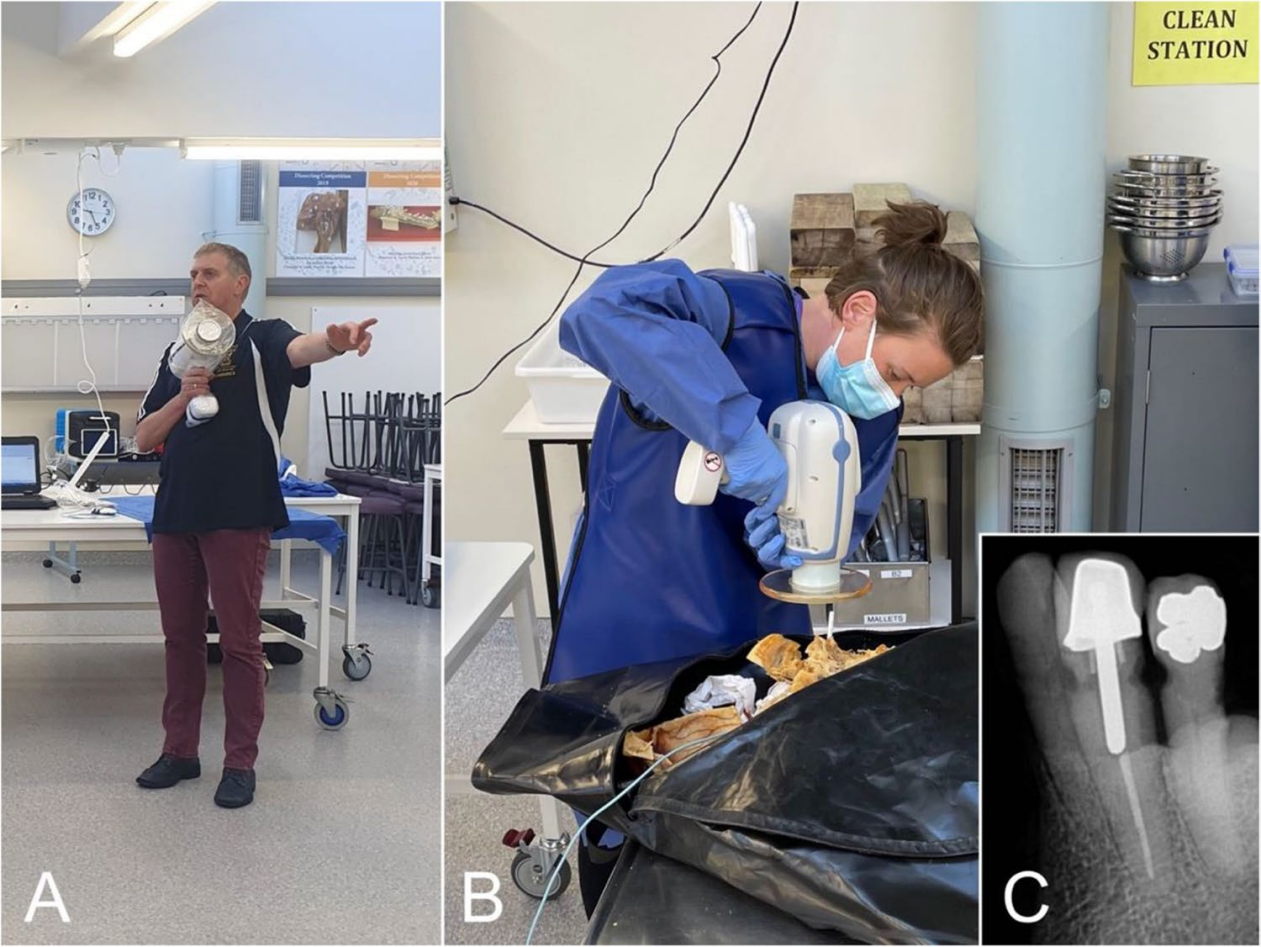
A The instructor introduces the participants to how to use the hand-held X-ray units (NOMADs) in a safe way. B A participant uses the NOMAD on embalmed human remains. C An X-ray image produced by the participants using the NOMAD device is depicted
